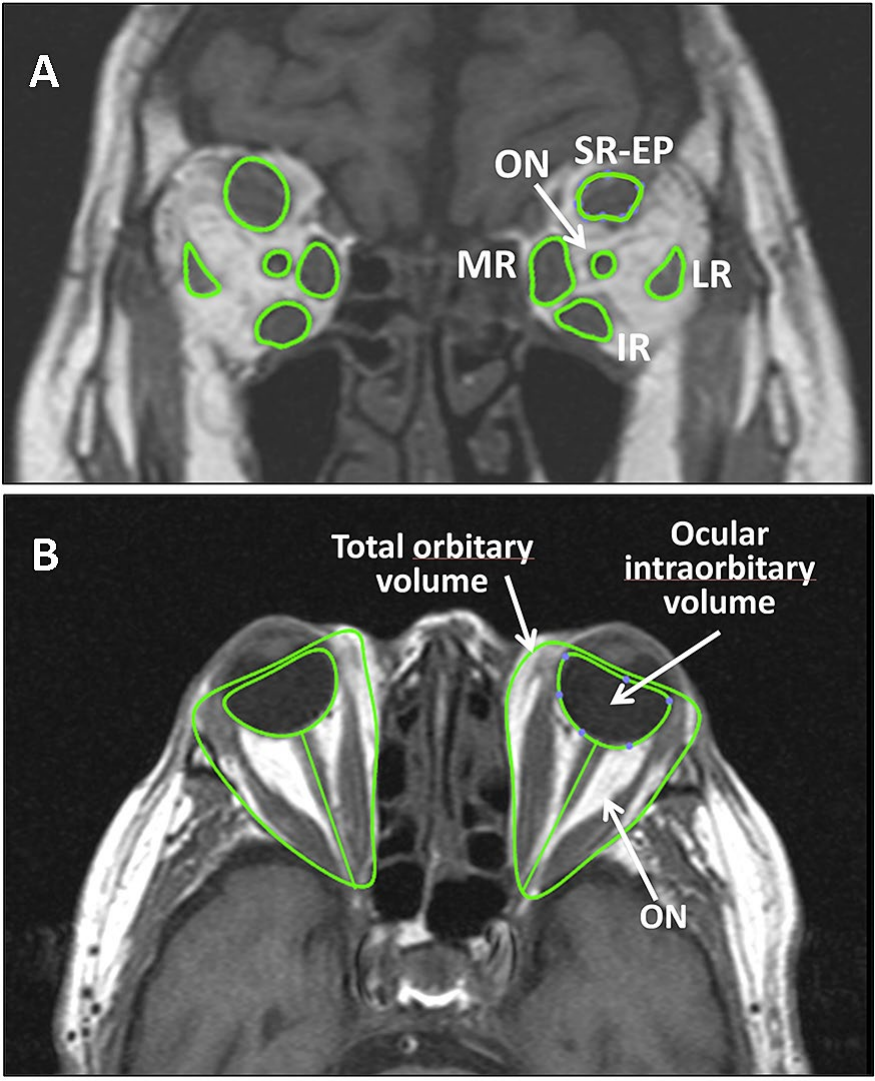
MRI image of a patient, in which the regions of interest are outlined. The images were transferred in DICOM format to Osirix Lite software in order to calculate the volume of the studied orbital structures. A: SR-EP: Superior rectus -Elevator of the eyelid complex; LR: Lateral rectus; IR: Inferior Rectus; MR: Medial Rectus; ON: Optic nerve. B: Delimitation of total orbitary volume and intraorbitary portion of the eyeball are indicated. The total orbital fat was assumed to be the total orbital volume after subtracting the intraorbital portion of the eyeball as well as the volume of extraocular muscles (SR-EP, LR, IR, MR) and optic nerve
